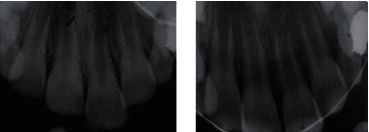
(a) Initial periapical radiograph. (b) Radiographic examination after *repositioning* is completed.
